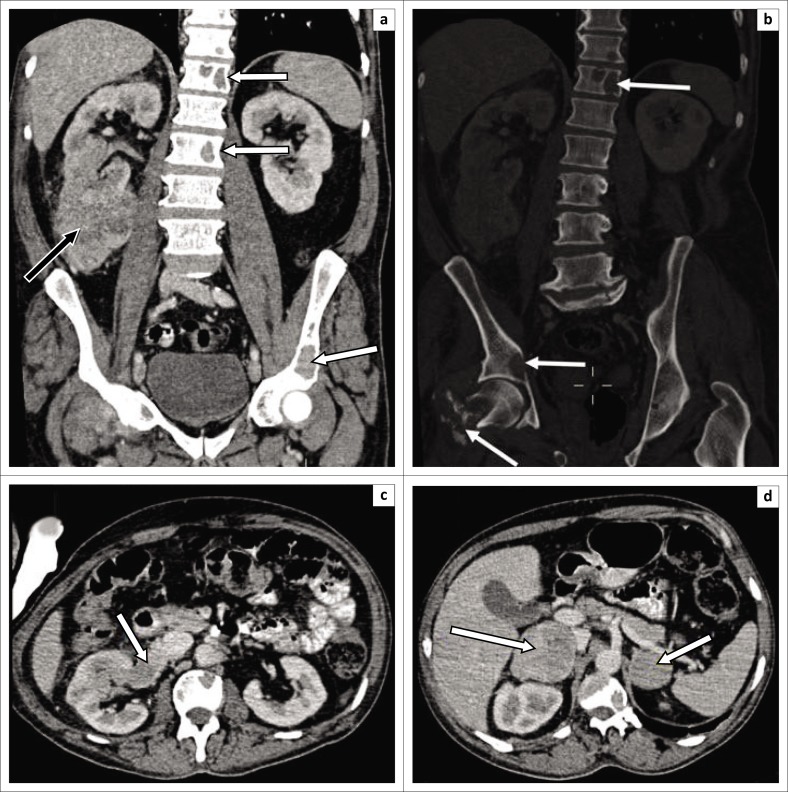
Renal cell carcinoma (RCC) in a patient who presented with a pathological right femoral neck fracture. Portovenous phase computed tomography scan coronal images demonstrate (a) a large exophytic heterogeneously enhancing right renal lower pole mass (black arrow) with perinephric fat stranding and thickening of Gerota’s fascia. (a and b) Multiple lytic skeletal metastases in the spine, pelvis and right femur with associated neck of femur fracture (white arrows). (c) An enhancing tumour thrombus in the right renal vein (arrow). There is no extension of the tumour thrombus to the inferior vena cava. (d) Bilateral adrenal gland metastases (arrows) were present. These findings are consistent with stage 4 disease.
